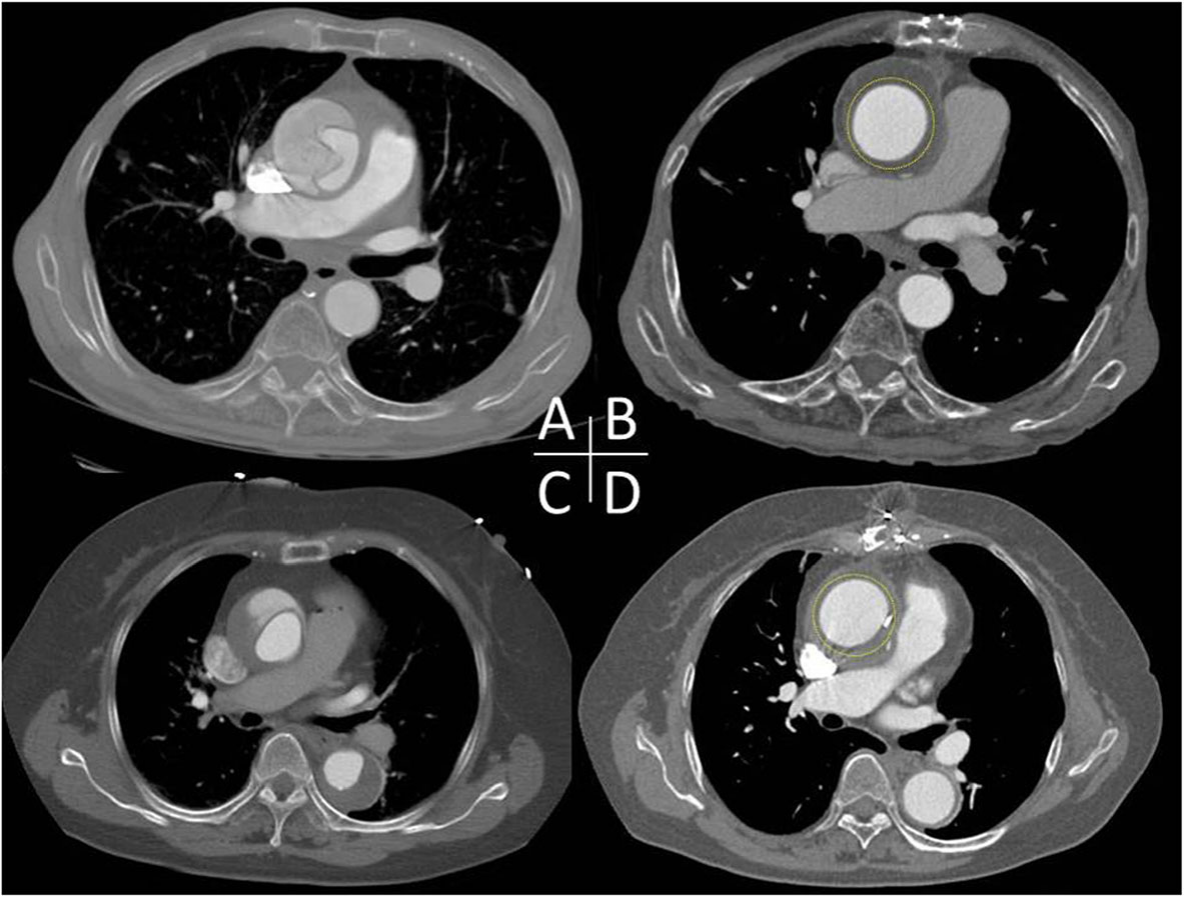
a and c Preoperative computed tomographic scan. b and d Follow-up postoperative computed tomographic scan after stepwise external wrapping procedure, demonstrating disappearance of false lumen and complete remodeling of the ascending aorta. Yellow dot showing line of the artificial graft
